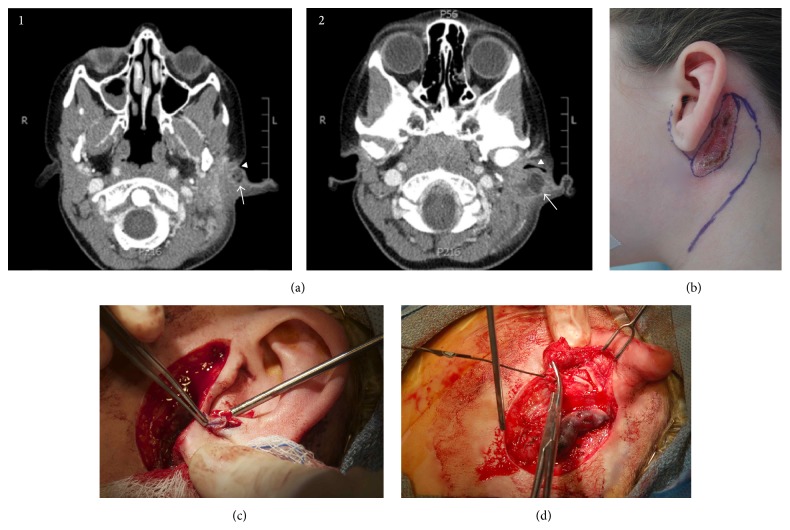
Case  5. (a)(1-2) CT scan with contrast, axial cuts demonstrating a left rim-enhancing postauricular abscess (arrow) adjacent to the conchal bowl (where puncta were identified at time of surgery), causing EAC stenosis (arrowhead). (b) Modified Blair incision incorporating postauricular granulation tissue (site of prior I&D). The limb of the marked incision extending down along a neck crease was marked as a possibility but not incised during the surgery, since it was unnecessary for visualization of the digastric and SCM muscles. (c) After identifying the facial nerve, an elliptical incision was carried out around the antitragal pit and dissected down. (d) Both the gentian violet within the tract and the lacrimal probe were used throughout the case to identify the tract. After initial dissection, the ellipsed skin around the pit and the contiguous tract was passed deep to the auricle and brought out through the postauricular incision keeping the entire tract intact.
